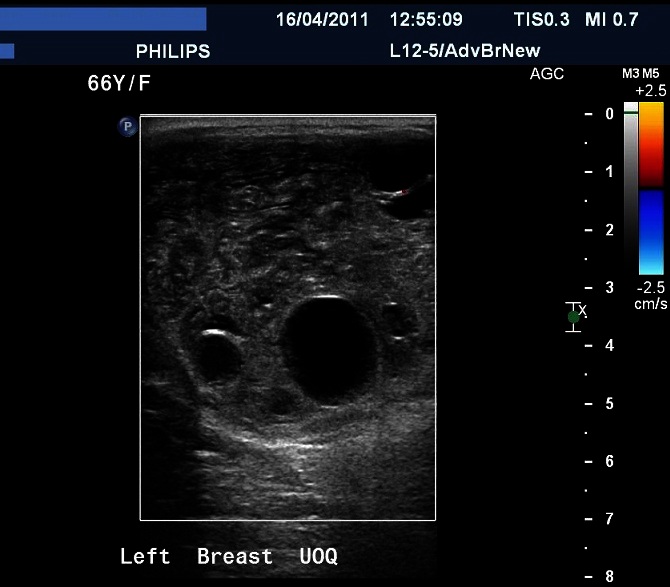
Doppler integrated image shows no internal flow within the mass
